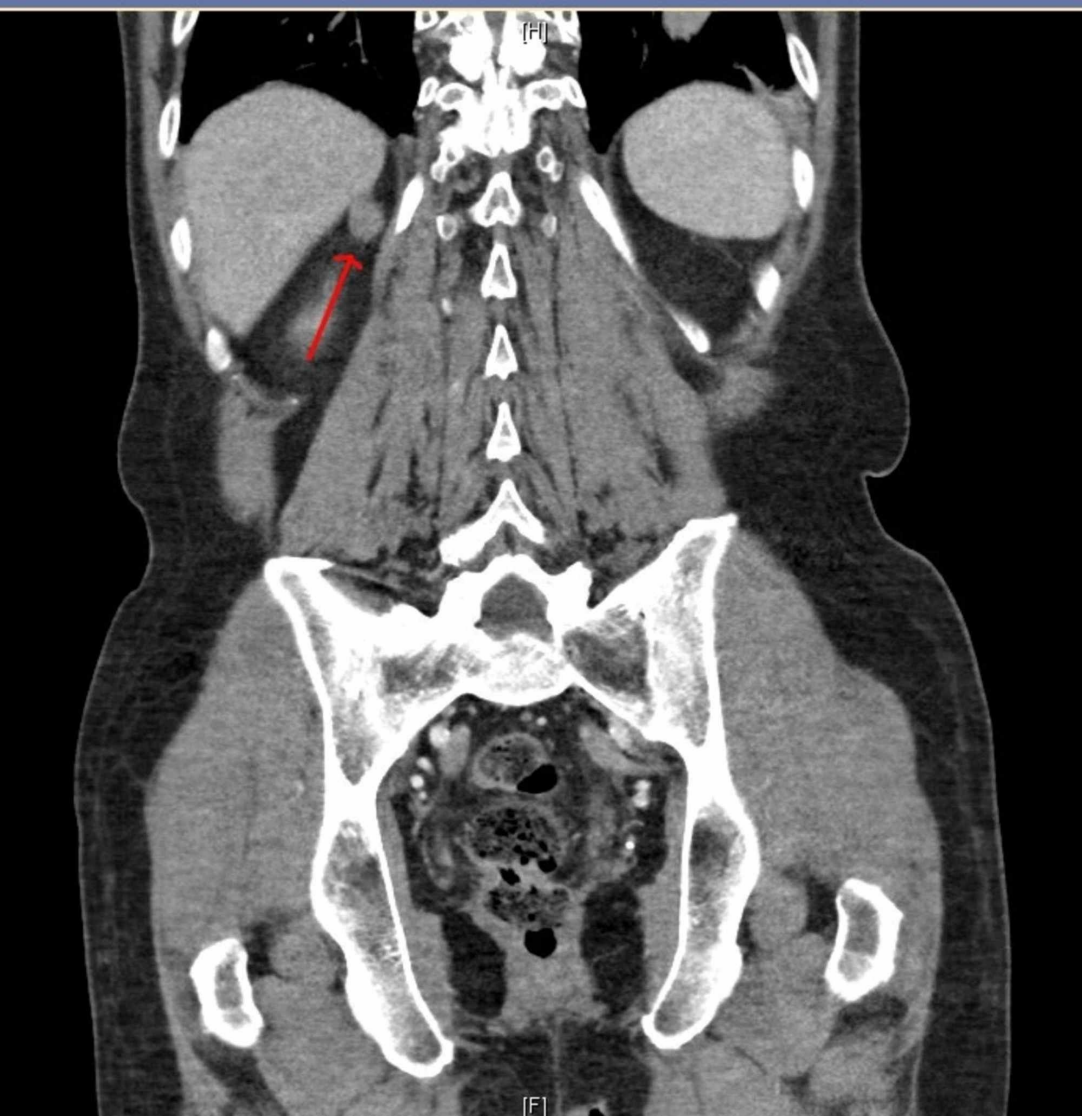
CT chest abdomen and pelvis
